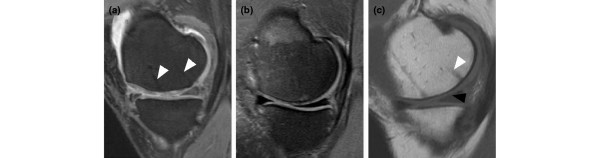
Magnetic resonance imaging (MRI) four years after implantation of BioSeed^®^-C. (a) Out of 17 patients, 11 patients, including this one, showed complete filling of the defect four years after implantation of BioSeed^®^-C. (b) Five patients, including this one, showed more than 50% defect filling but not complete defect filling. (c) One patient showed less than 50% defect filling (black arrowhead). The repair tissue gives a slightly altered MRI signal, and transosseous drill holes are still evident (white arrowheads).
